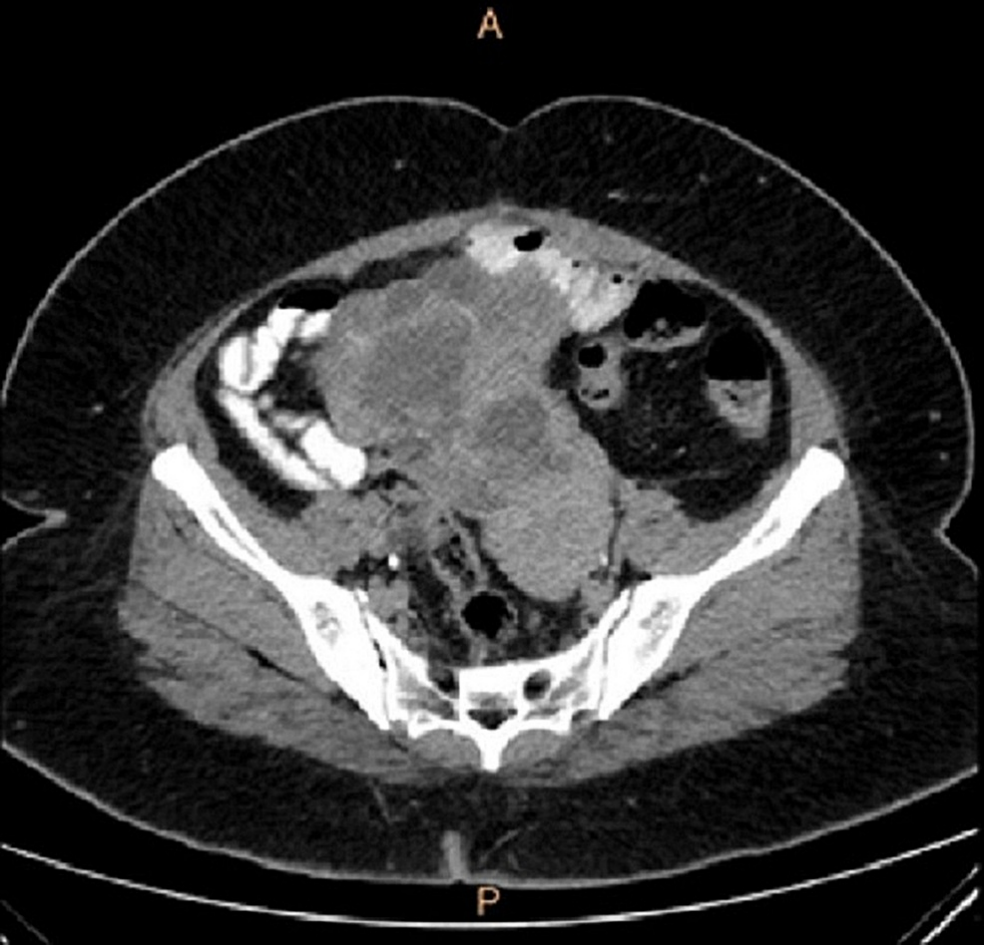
CT image showing lobulated heterogeneously enhancing complex cystic mass in the right adnexa
